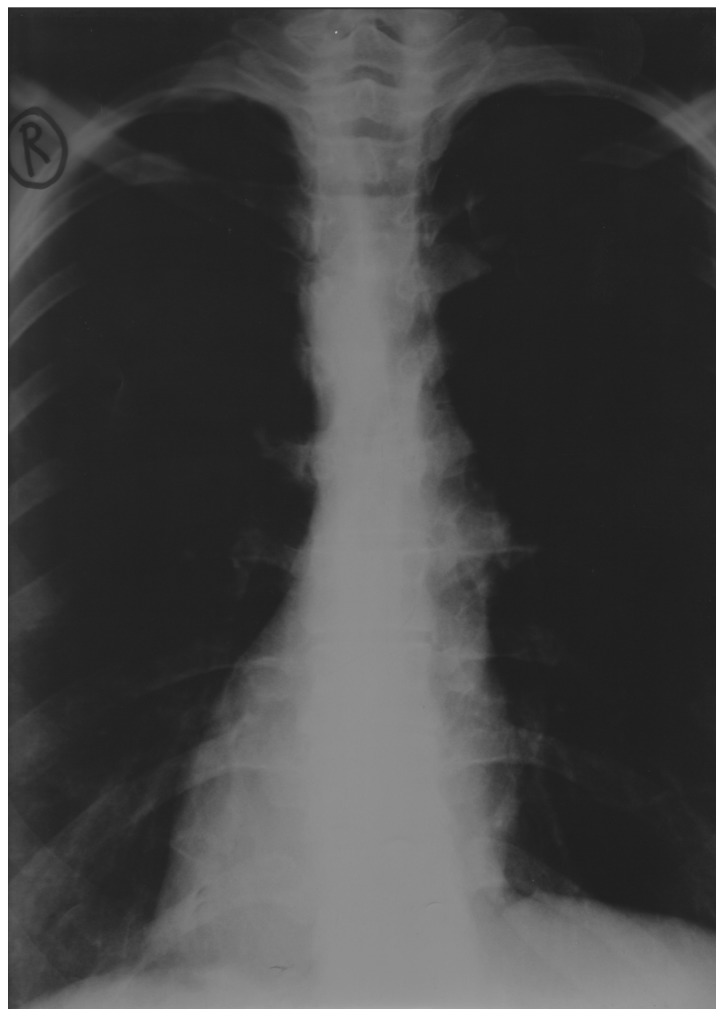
Chest radiography of the patient. Dextrocardia and a right subphrenic gastric bubble are evident.
